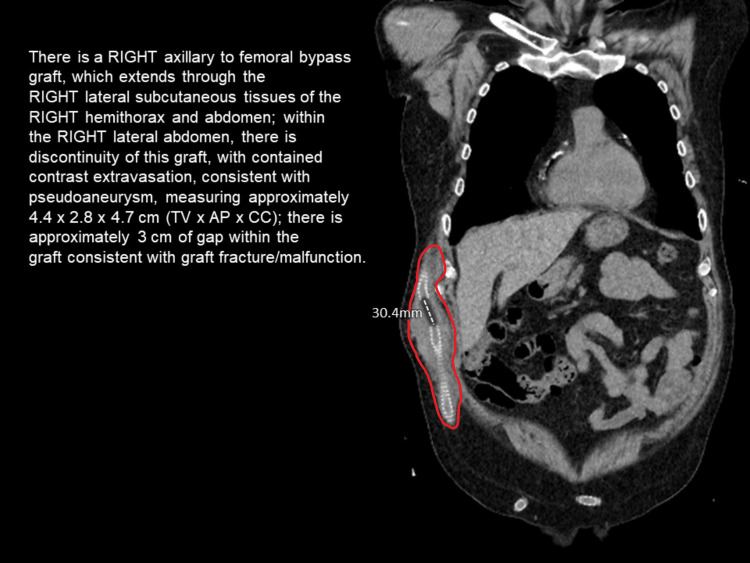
CT angiogram showing graft fracture with pseudoaneurysm and active extravasation. The red outline indicates the boundaries of the pseudoaneurysm.
